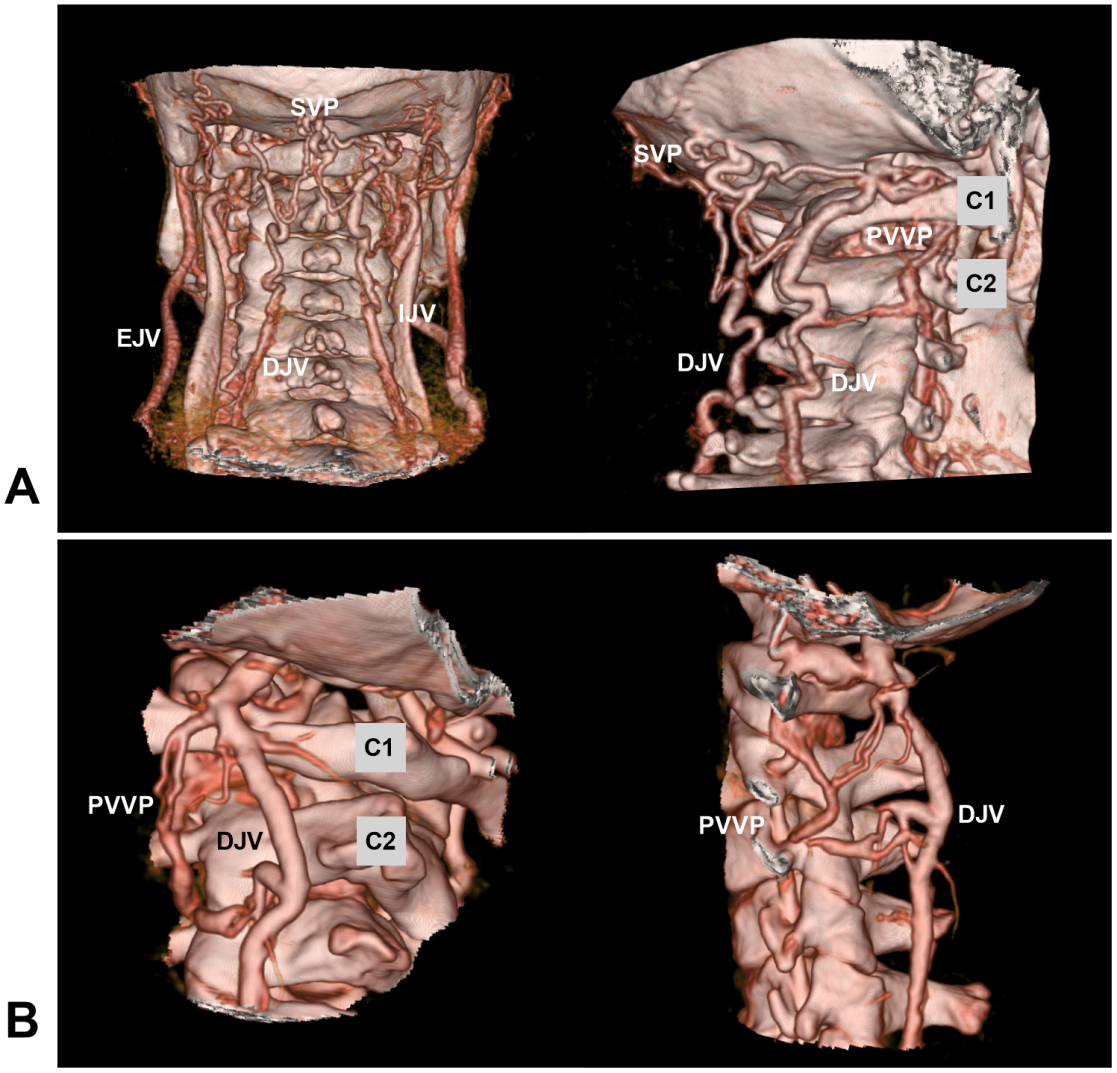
Venous plexuses in the CCJ region. (A) Posterior anterior view (left panel) and oblique view (right panel) CTA showing the anastomosed SVP, PVVP, and DJV. (B) Oblique view (left panel) and lateral view (right panel) CTA showing the anastomosed PVVP and DJV. C1, C2, first and second cervical vertebrae; CCJ, craniocervical junction; CTA, computed tomography angiography; DJV, deep jugular vein; EJV, external jugular vein; IJV, internal jugular vein; PVVP, paravertebral venous plexus; SVP, suboccipital venous plexus.
